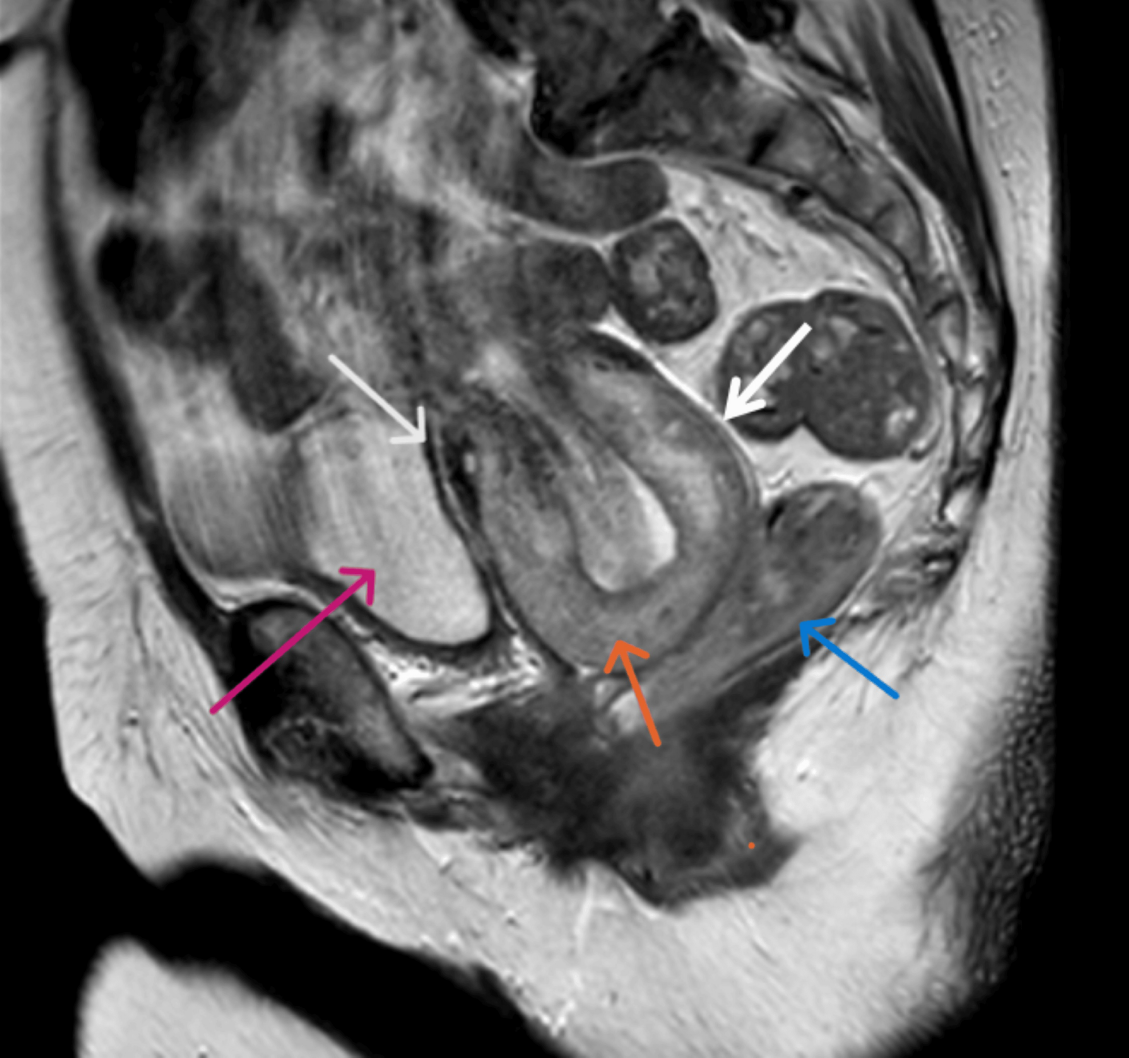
MRI characteristics of uterine inversion in T2-sagittal view. The white arrows point to the anterior and posterior vaginal walls, distended by the uterine corpus; the orange arrow points to the uterine fundus; the pink arrow points to the bladder; the blue arrow points to the rectum.
